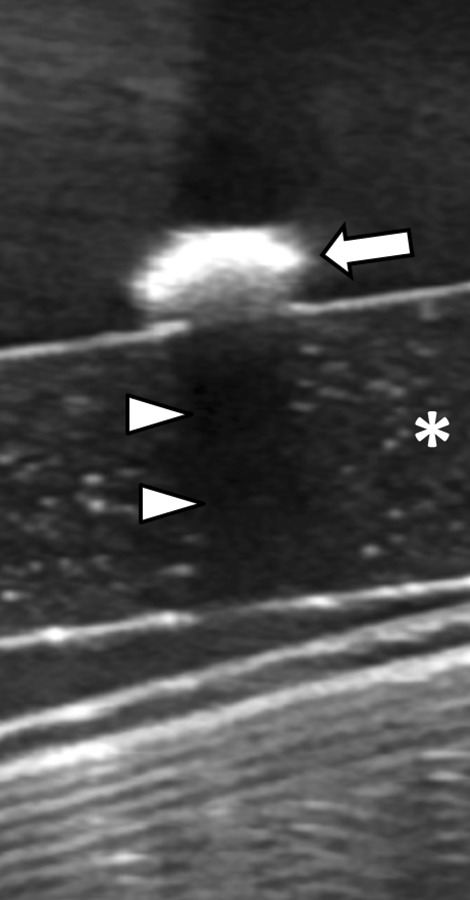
In vitro ultrasound of xanthine urinary stone: stone (arrow) is imaged in a water bath on a standoff pad (asterisk) and is echogenic with posterior acoustic shadowing (arrowheads)
